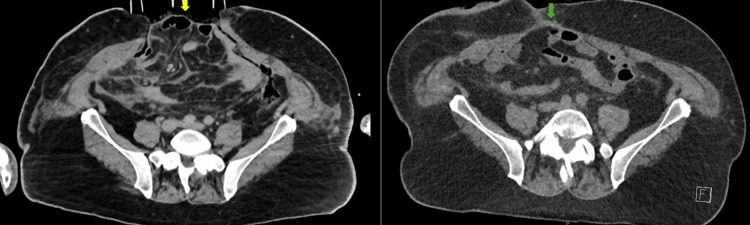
Axial CT abdomen pelvis (left) indicated a fascia defect (yellow arrow) on day 1 post application of the Fasciotens. CT abdomen pelvis (right) indicated successful primary fascia closure (green arrow) prior to discharge.
